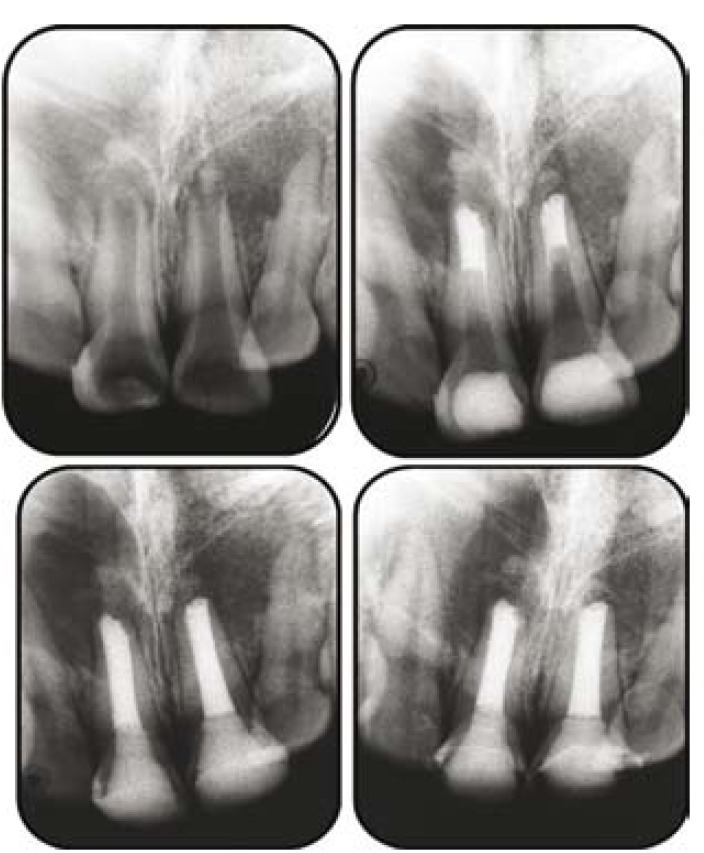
a) Preoperative radiograph; b) MTA plug; c) Post obturation radiograph; d) Healing eident after 3 months.
